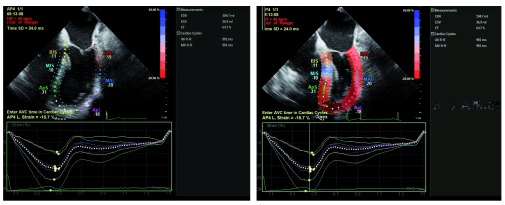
Post-processing of the two-dimensional echocardiographic images with strain software allows assessment of the motion and strain of the heart throughout the cardiac cycle (left, diastole) (right, systole). ApL, apical lateral; ApS, apical septal; BAL, basal anterolateral; BIS, basal inferoseptal; bpm, beats per minute; HR, heart rate; MAL, mid anterolateral; MIS, mid inferoseptal; SD, standard deviation; AVC, aortic valve closure; AVR-R, aortic valve R-R interval; EDV, end-diastolic volume; EF, ejection fraction; ESV, end-systolic volume; MVR-R, mitral valve R-R interval.
